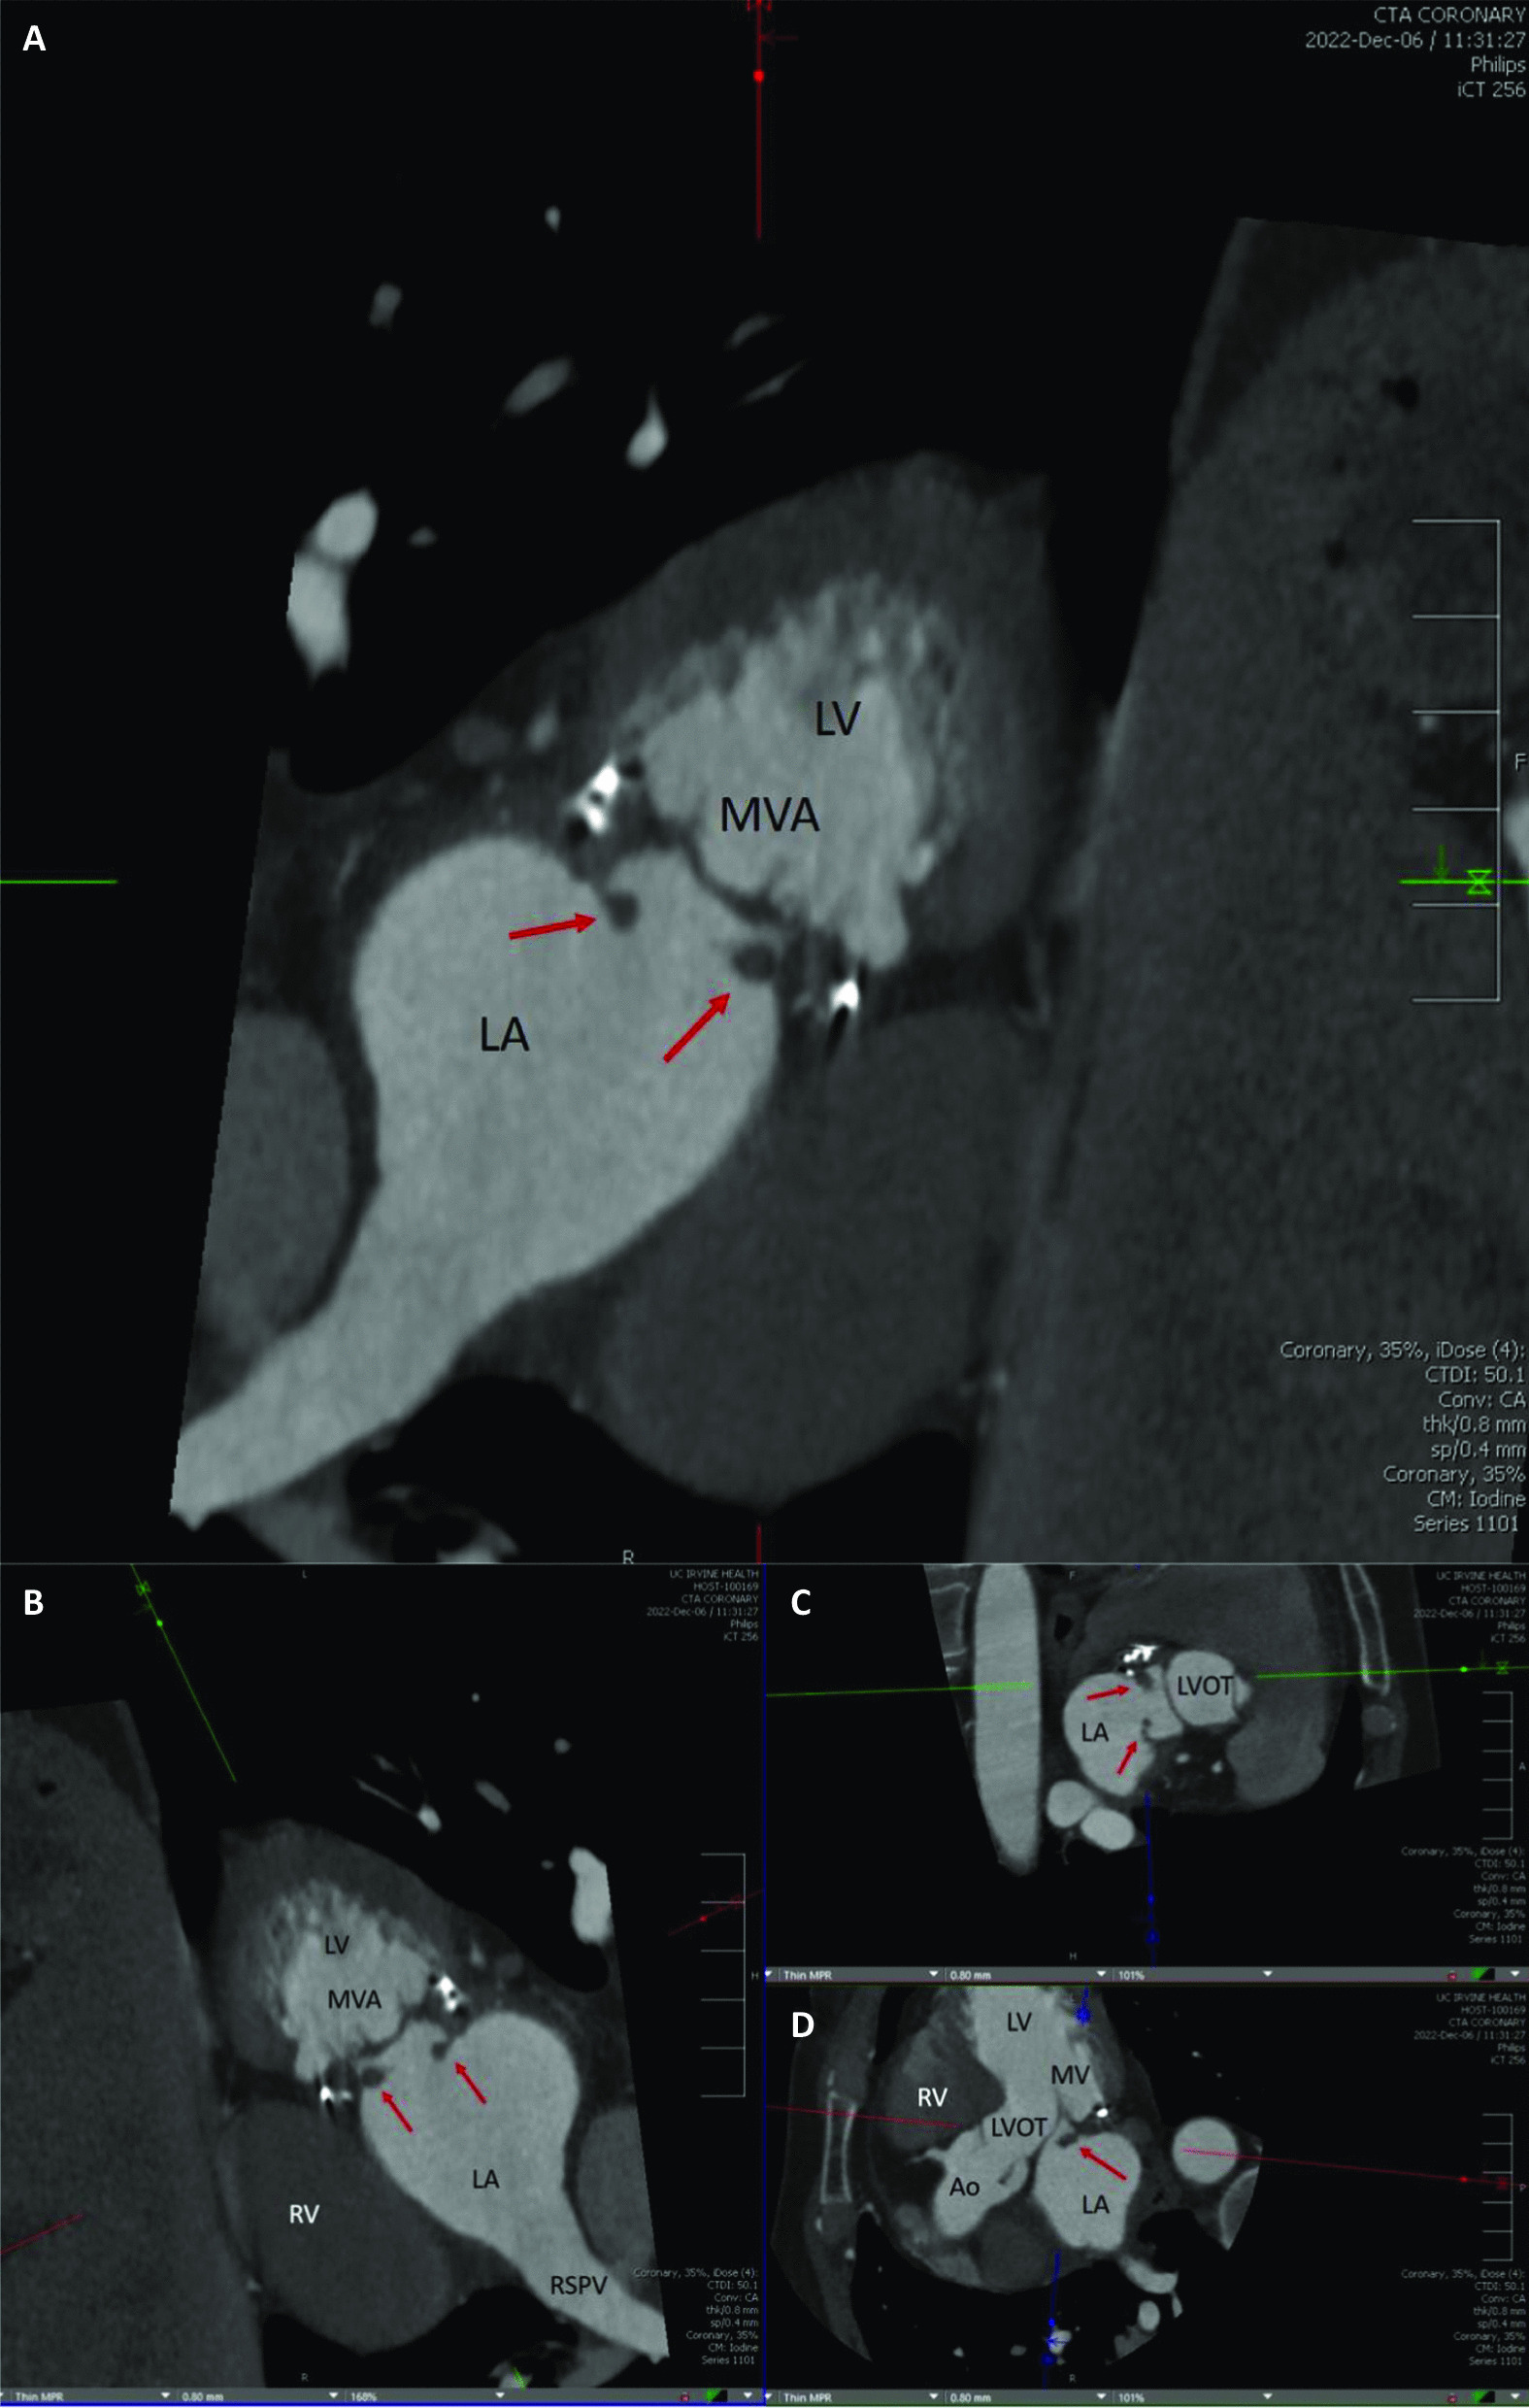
Computed tomography coronary angiogram. A Computed tomography coronary angiography of the mitral valve annulus demonstrating two filling defects, representing endocarditis (red arrows). B A multiplanar computed tomography coronary angiogram array showing filling defects in the left atrium. The labeled structures include the left ventricle, right ventricle, right superior pulmonary vein, aortic root, left ventricular outflow tract, and coaptation of the mitral valve leaflets. C, D Provide additional views
